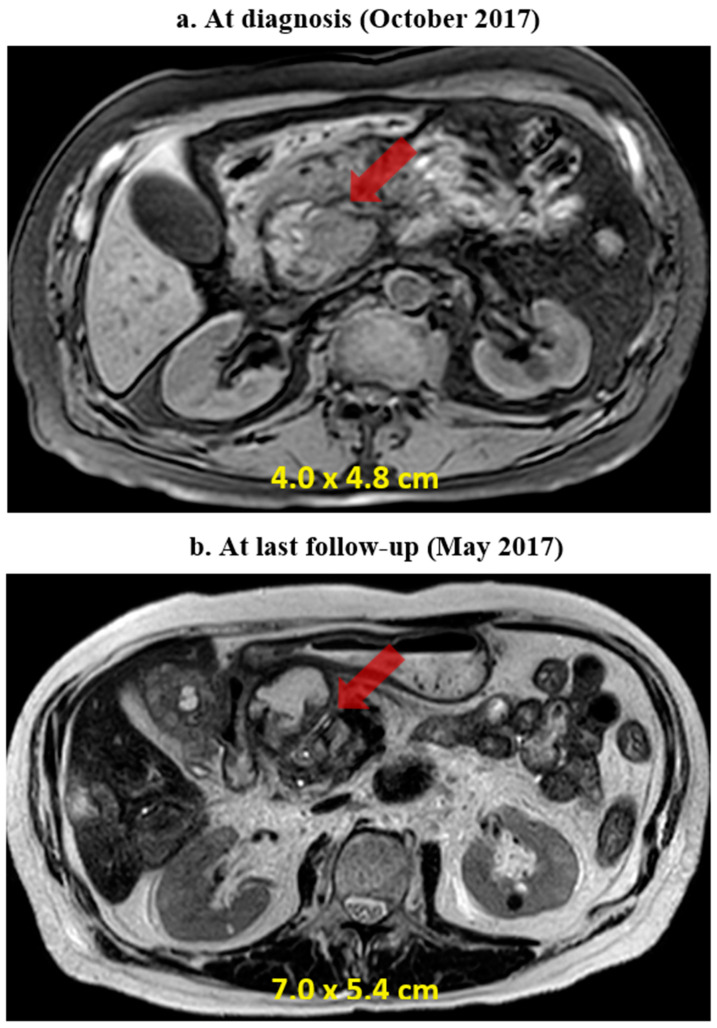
Comparative magnetic resonance imaging (MRI) of the abdomen (a) at diagnosis (October 2017) and (b) at the last follow-up (May 2018), showing disease progression despite several lines of treatment. The arrow indicates the pancreatic tumor.
